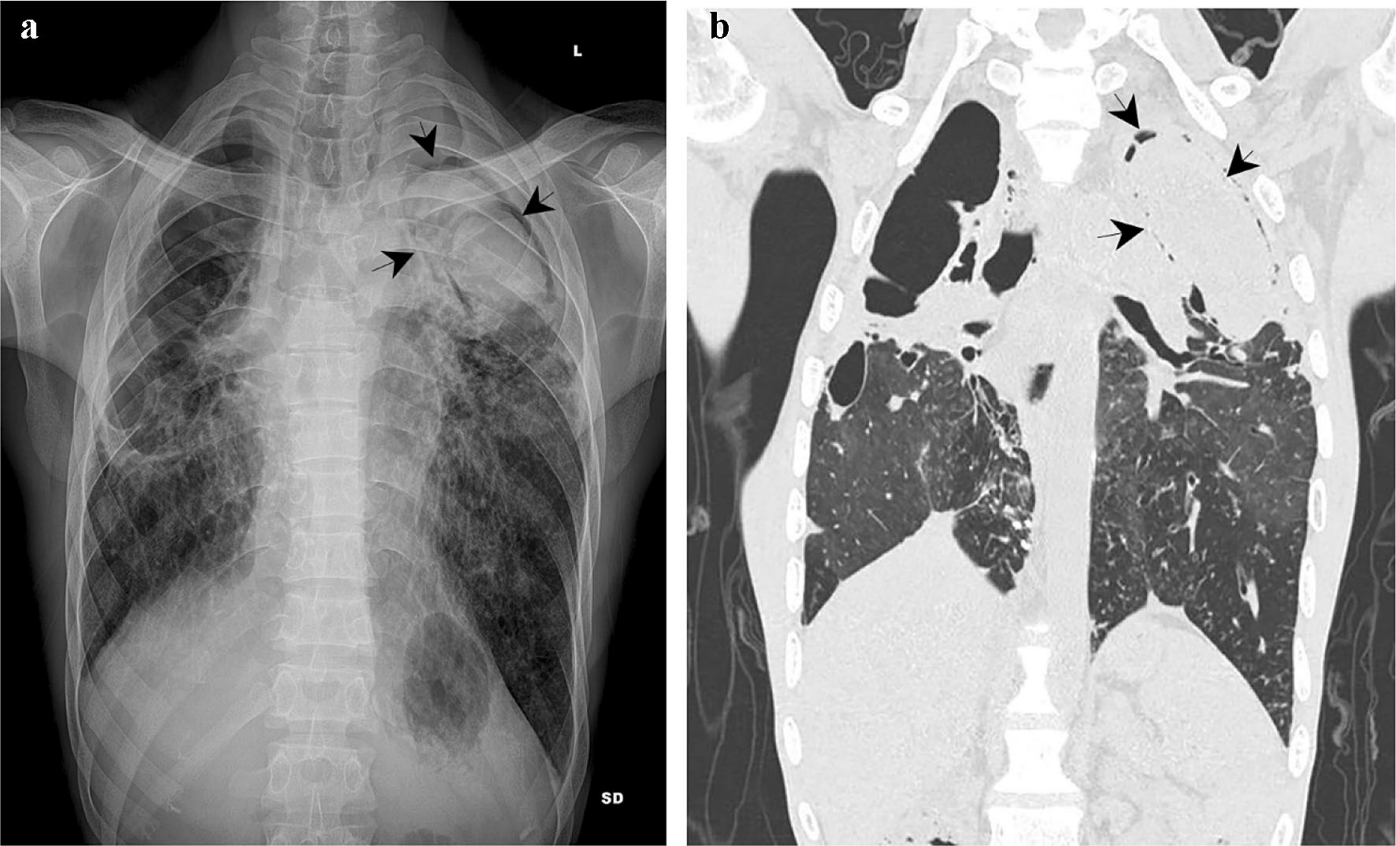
Posterior-anterior radiograph of the chest (a) and coronal CT reformation (b) shows fibrocavitary lesions in bilateral upper lobes. Left upper lobe cavity shows dependant intracavitary opacity with air crescent (arrow) in keeping with aspergilloma
